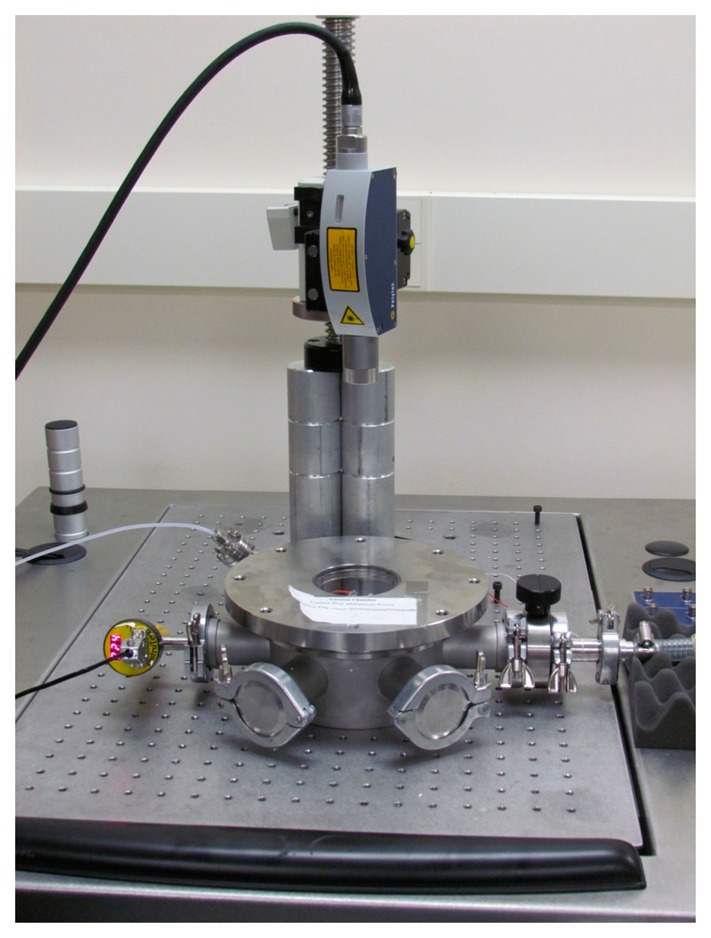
A picture of the experimental set-up used for testing the MEMS capacitive accelerometer showing the laser Doppler vibrometer and the vacuum chamber.
